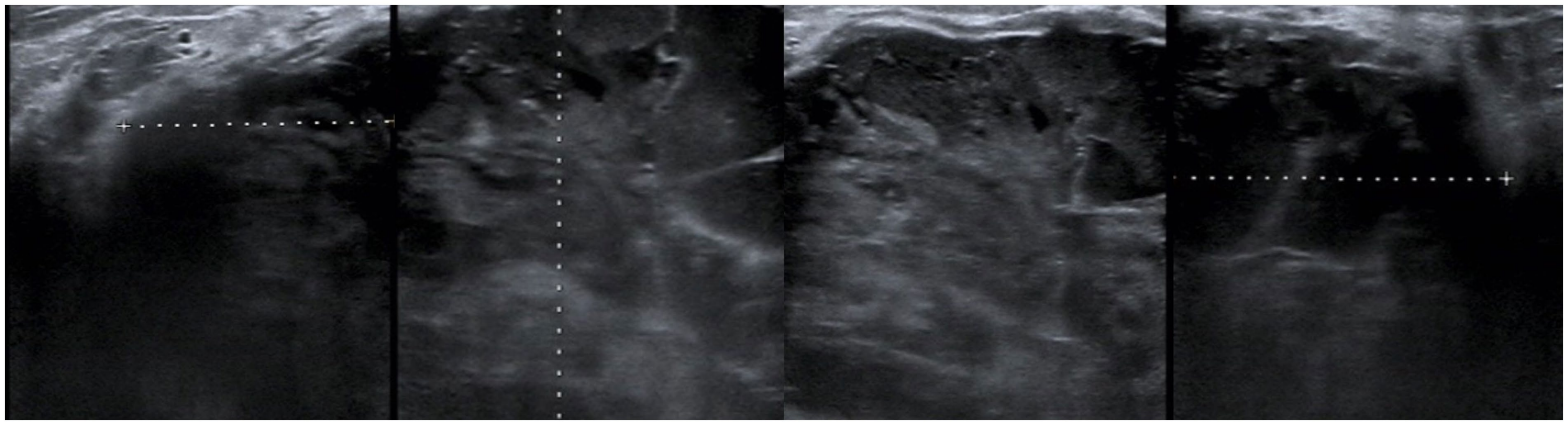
Ultrasound image from September 15, 2022. A hypoechoic mass measuring 154.2*66.1 mm is visible in the right breast, with indistinct borders and an irregular shape.
